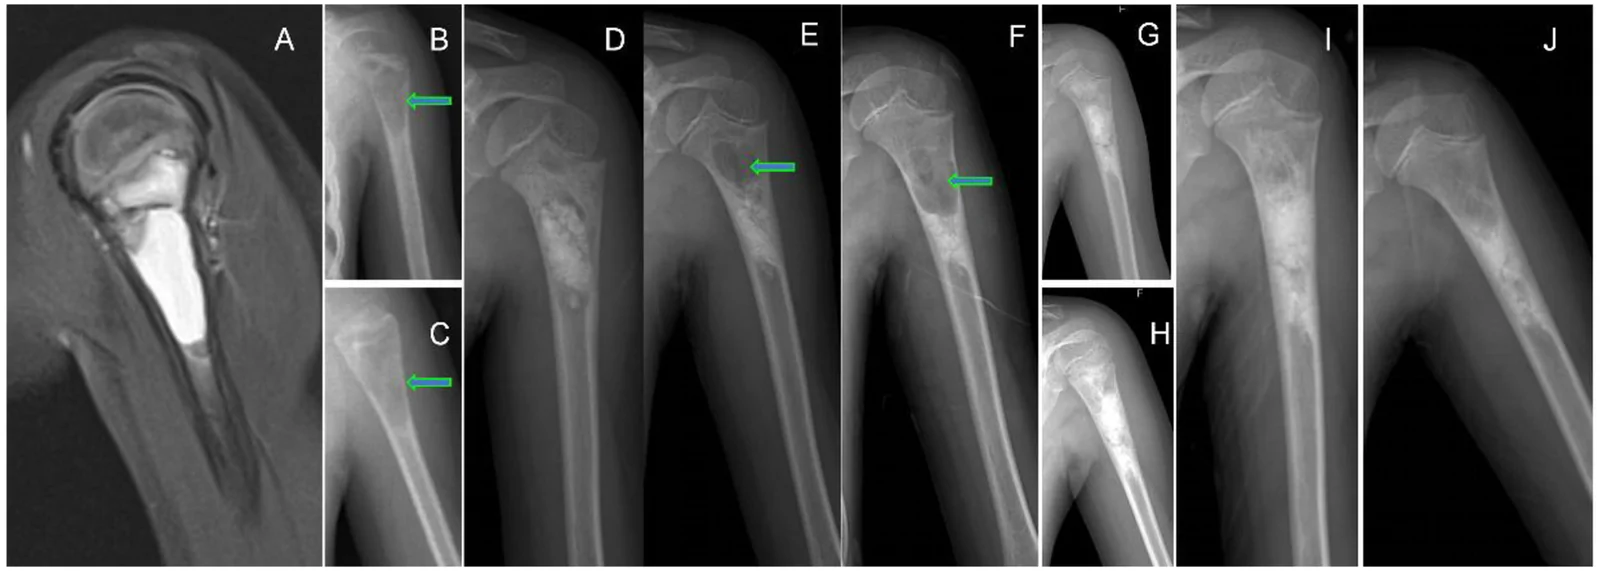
Imaging follow-up of a unicameral bone cyst treated with repeated percutaneous calcium phosphate cement injection. Initial MRI and radiographs showed a proximal humeral cystic lesion (A–C). Follow-up radiographs at 3, 12, and 24 months after the first injection demonstrated progressive cyst filling and remodeling (D–F). After a second injection (G,H), radiographs at 12 and 24 months showed further consolidation without obvious progression (I,J).
